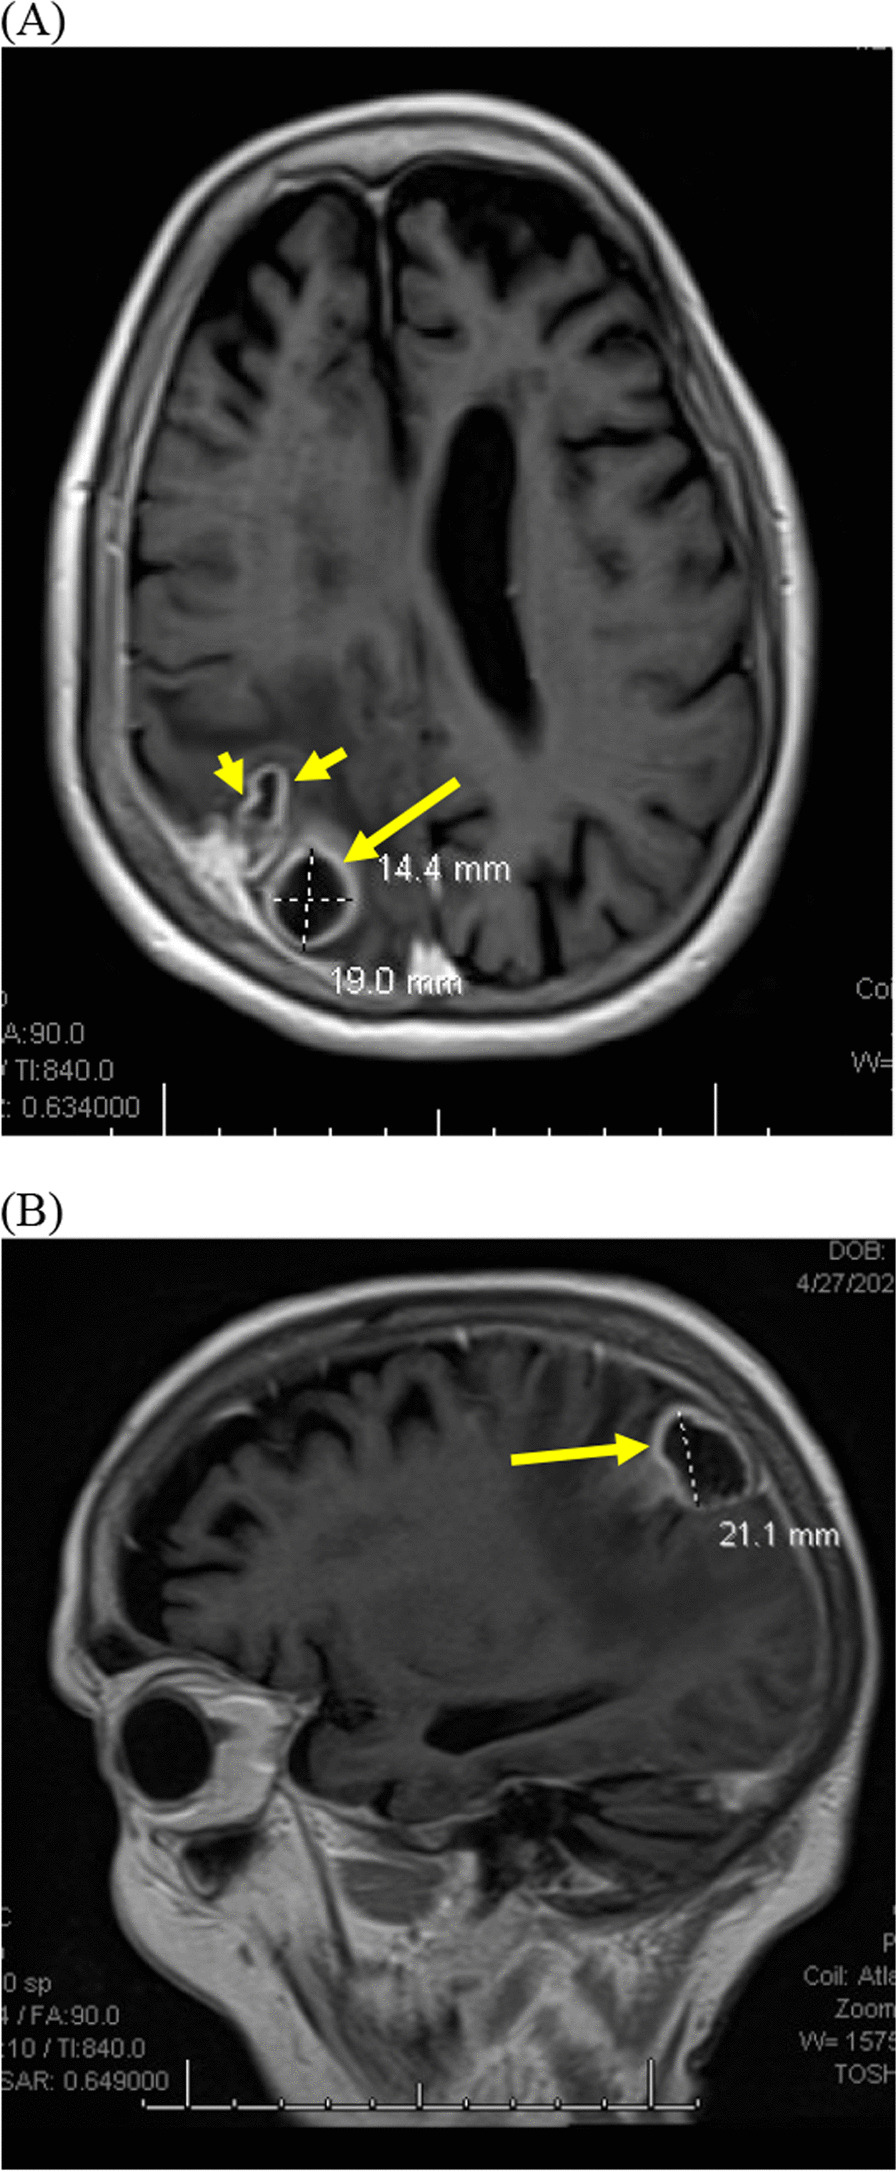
Brain magnetic resonance imaging images in transverse (A) and sagittal (B) views demonstrate multiple enhancing lesions (short yellow arrows) with vasogenic edema in the right parietal lobe consistent with abscesses, with the largest abscess measuring 1.4 cm × 1.9 cm × 2.1 cm (long yellow arrow)
